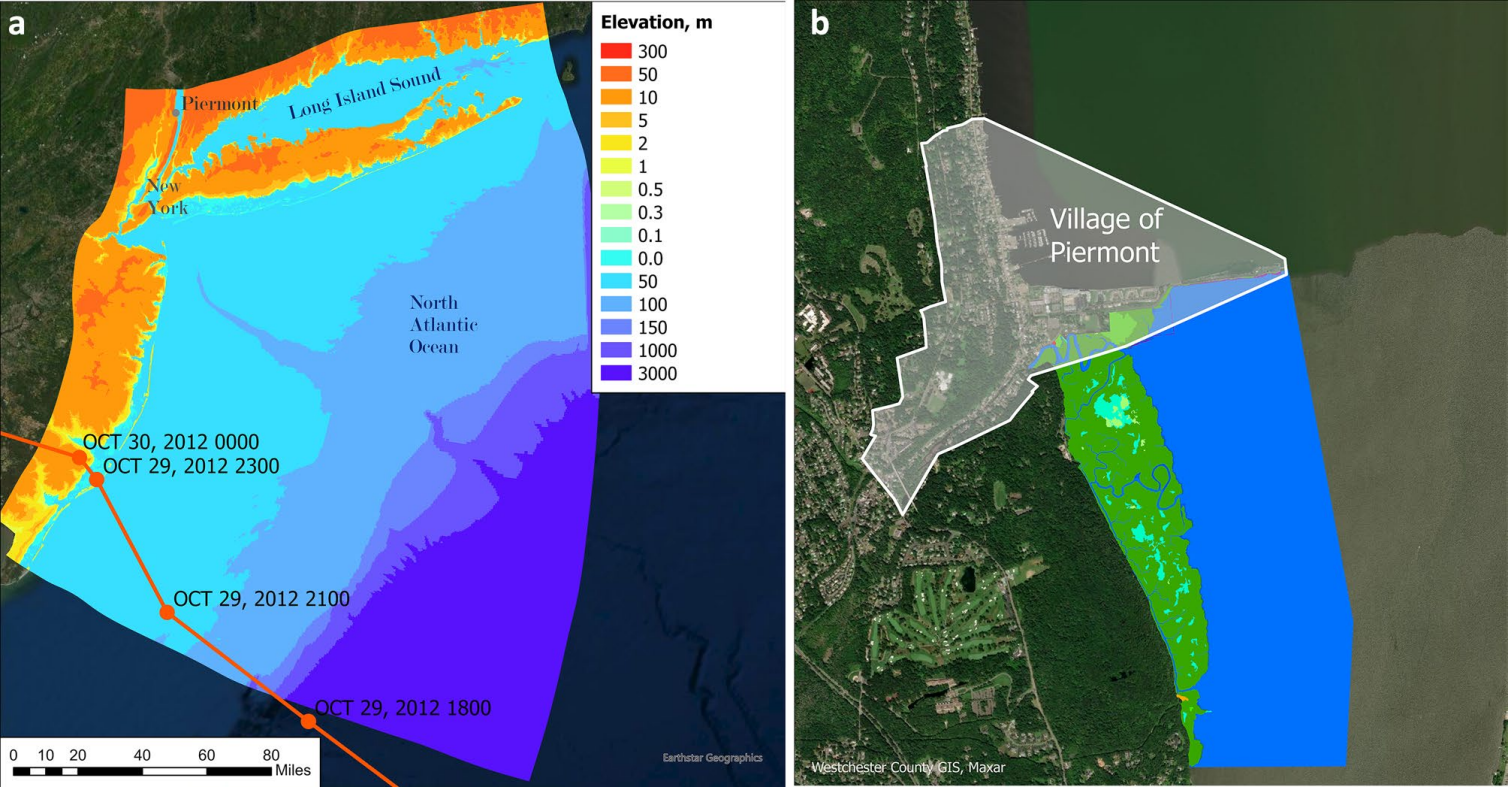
(a) NJ/NY/CT coastal domain and Hurricane Sandy (October 2012) track (left) (ArcGIS basemap credit: Earthstar Geographics). (b) Piermont Village and Marsh (right) (ArcGIS basemap credit: Westchester County GIS, Maxar).
